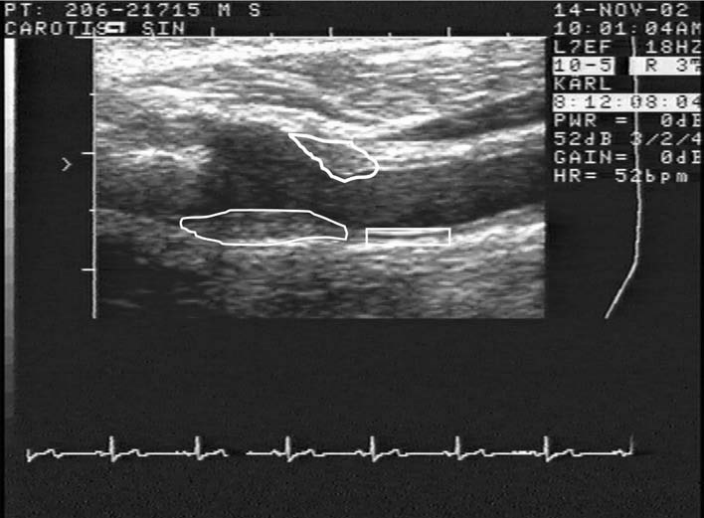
An ultrasonographic image of the left carotid artery from a study participant. The blood inside the artery appears black. The right side of the image shows the part of the common carotid artery where IMT and IM-GSM are measured (rectangle). IMT is the thickness of that part of the vascular wall, and IM-GSM is a measure of the echogenicity (gray scale) of the same part of the wall. At the left side of the image, the carotid artery has divided into an internal part and an external part. In the bifurcation region, two plaques are outlined.
